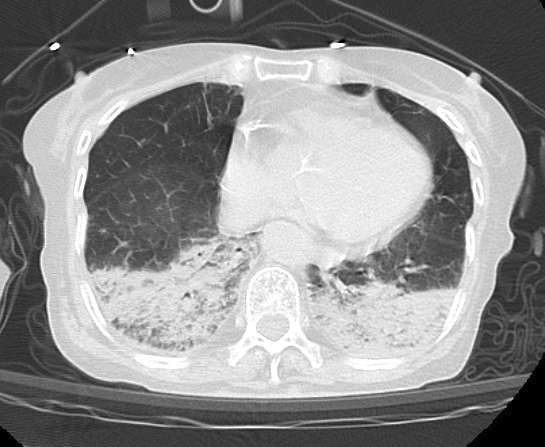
Chest computed tomography of the patient during admission revealed posterior atelectasis in both lungs, possibly due to increased airway secretions.
